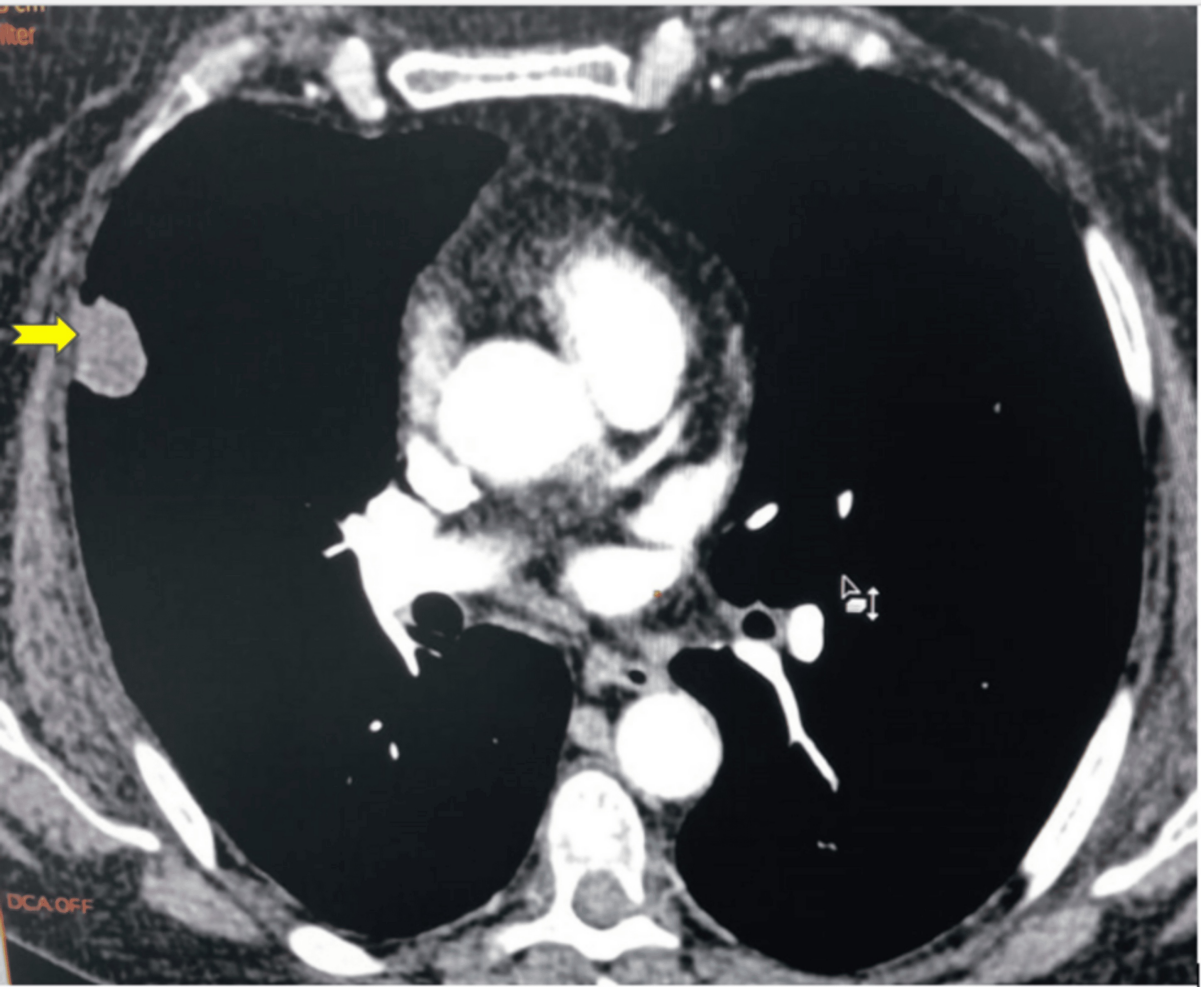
A thoracic CT scan with mediastinal window after injection of contrast medium showed the presence of a middle lobar parenchymal nodule. Yellow arrow: Middle lobar parenchymal nodule with regular contours, measuring 20 × 17 mm.
